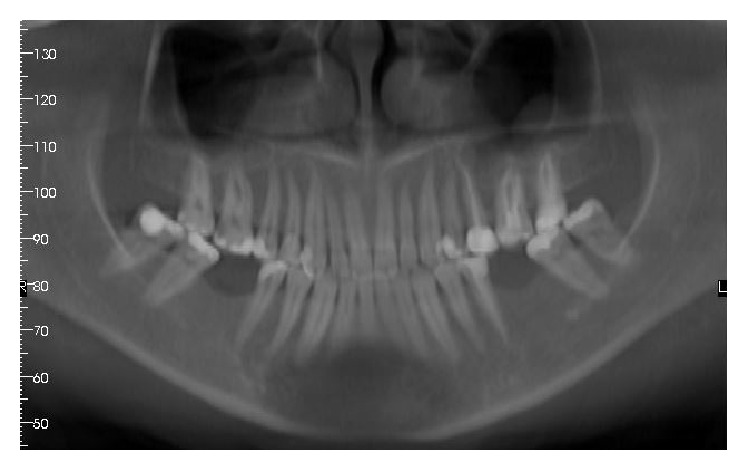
Panoramic X-ray showing teeth loss on the lower 1st molar area.
